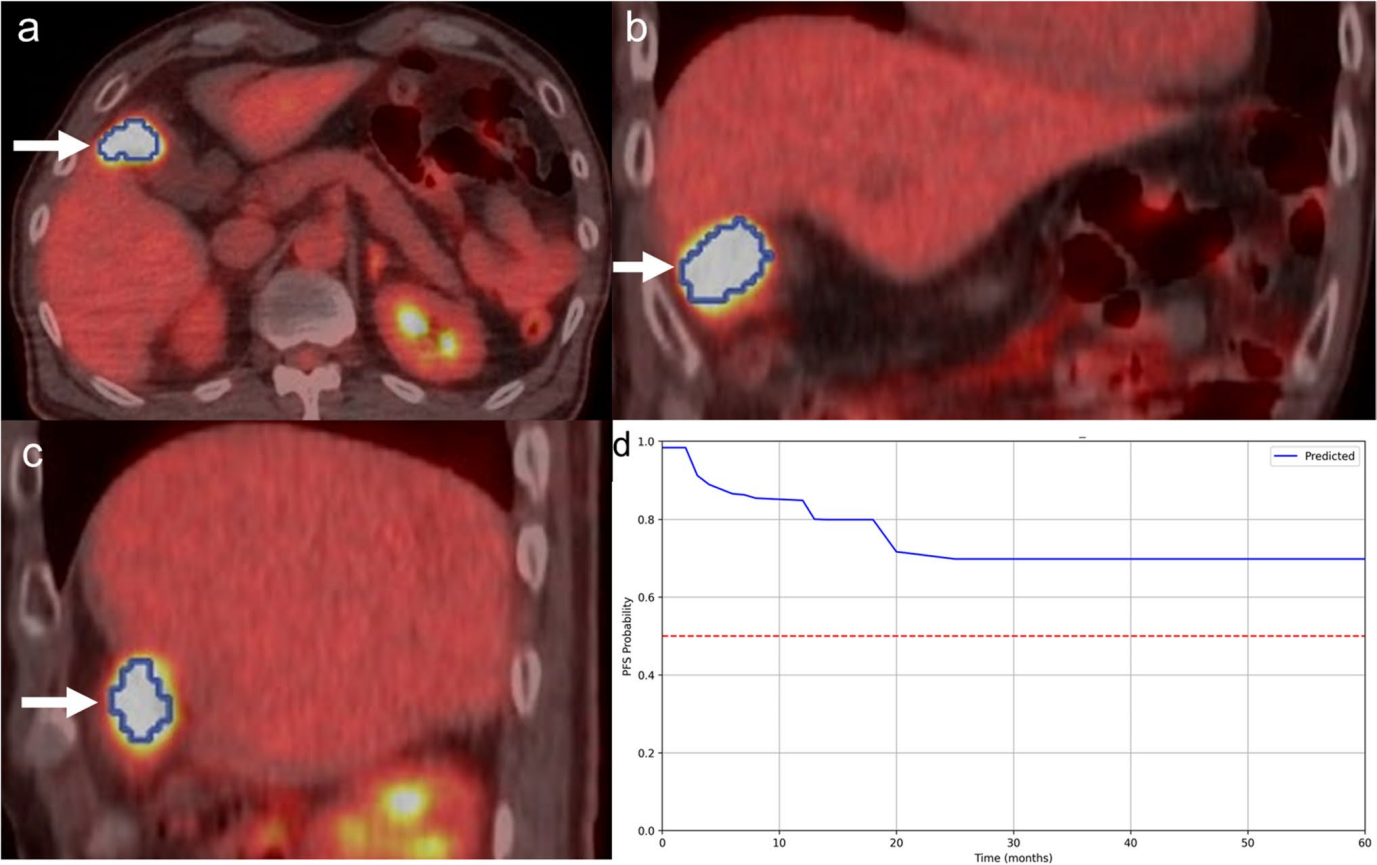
A 67-year-old man with gallbladder cancer (adenocarcinoma, T2N0M0, stage II) had no progression after surgery. Pretreatment [^18^F]-FDG-PET/CT [transaxial (a), coronal (b), and sagittal (c)] images revealed abnormal [^18^F]-FDG uptake in the primary lesion. The blue line represents the border of the VOI (arrows). The personalized survival prediction using the selected features ML model by the statistical feature selection method with the RSF algorithm (the best performing ML algorithm) is presented (d). The X-axis represents the survival time in months, and the Y-axis indicates the PFS probability. The PFS probability was 69.8% at 50 months after the surgery. The PFS probability did not reach 50%, and the ML algorithm predicted non-progression at this time. Indeed, the patient survived without progression for 50 months after the surgery
